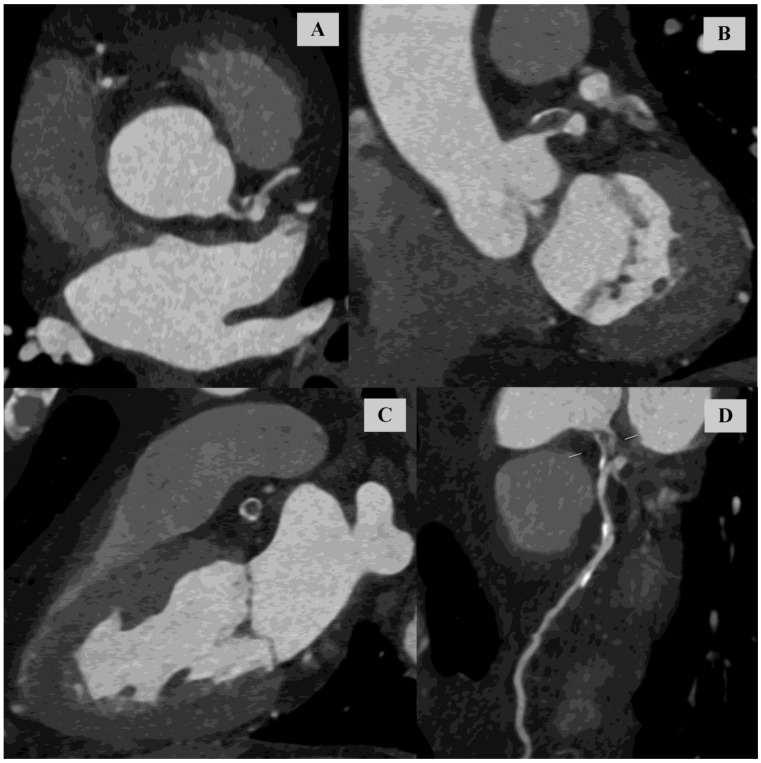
Coronary computed tomography angiography. Angiographic phase. (A) Multiplanar reconstruction (MPR) of the left main (LM) in a quasi-axial view, parallel to the long axis of the LM. (B) MPR of the LM in a quasi-coronal view, parallel to the long axis of the LM. (C) MPR of the LM in an oblique view, perpendicular to the long axis of the LM. (D) Curved multiplanar reconstruction (cMPR) of the left main (LM) extending into the left anterior descending (LAD). The gate (white dashed line) indicates the level of maximum LM stenosis.
